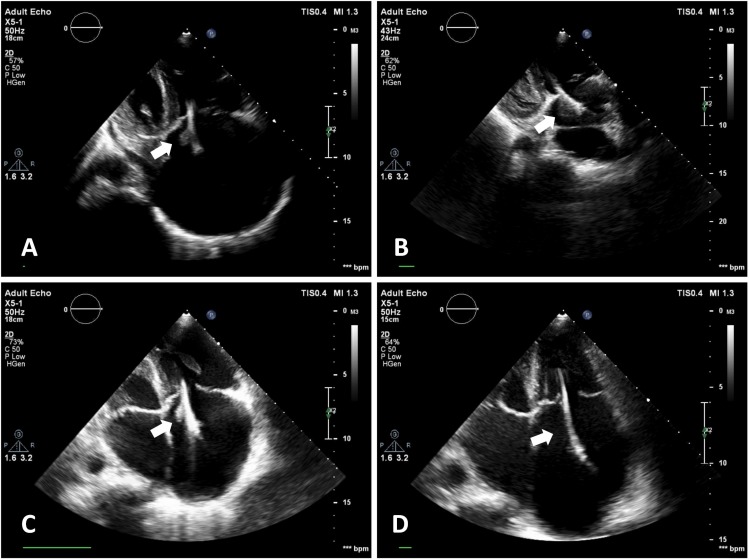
Transthoracic echocardiographic images from the apical 4-chamber view show the stages of the thrombus by time. The thrombus was completely resolved after the initiation of warfarin.
